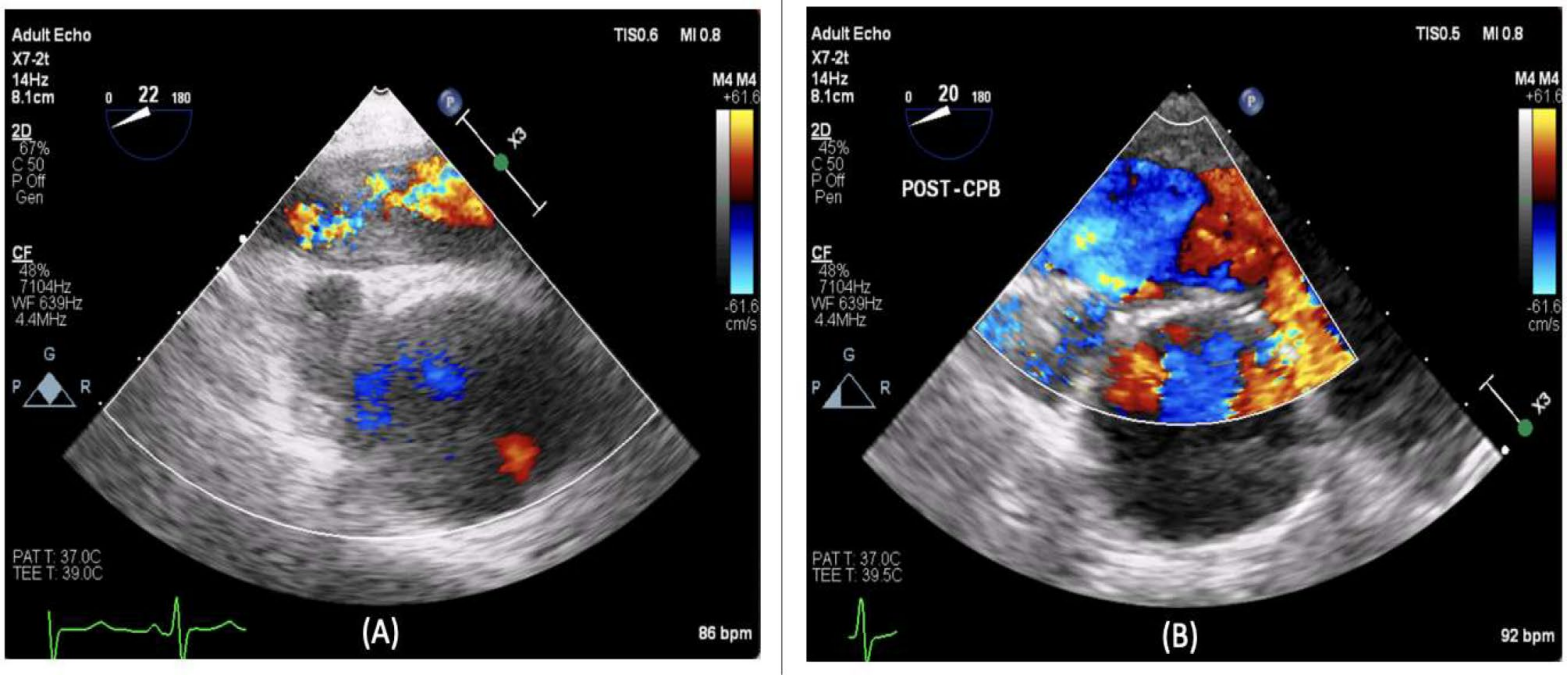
Shows a transoesophageal (Mid oesophageal, short axis, ascending aorta, colour flow doppler) (a) is preclearance and (b) is post clearance
